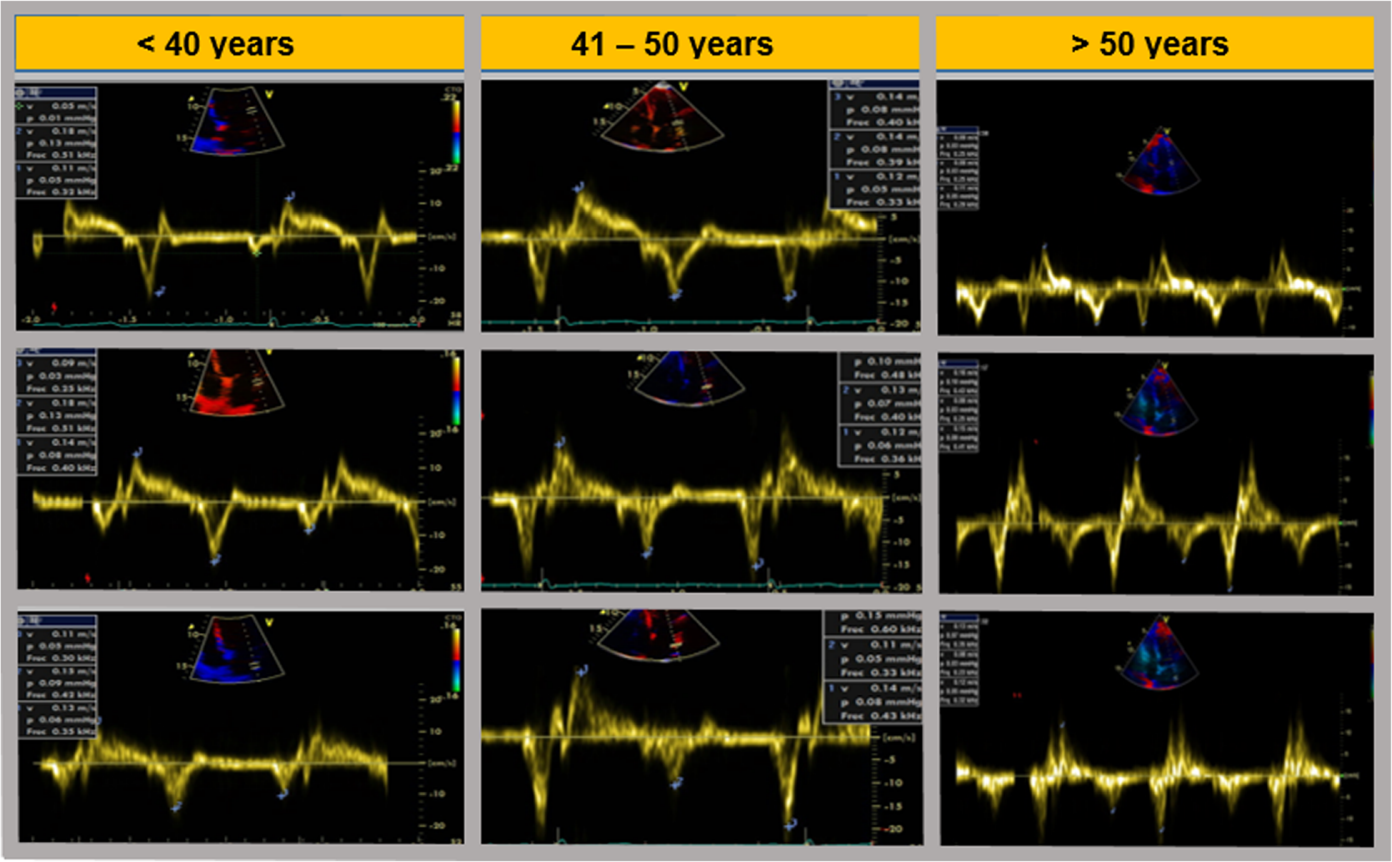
Pulsed TDI at the annular level (upper row), basal LA (middle row), and mid LA (lower row), according to age groups. TDI: Tissue Doppler Imaging, LA: left atrial
